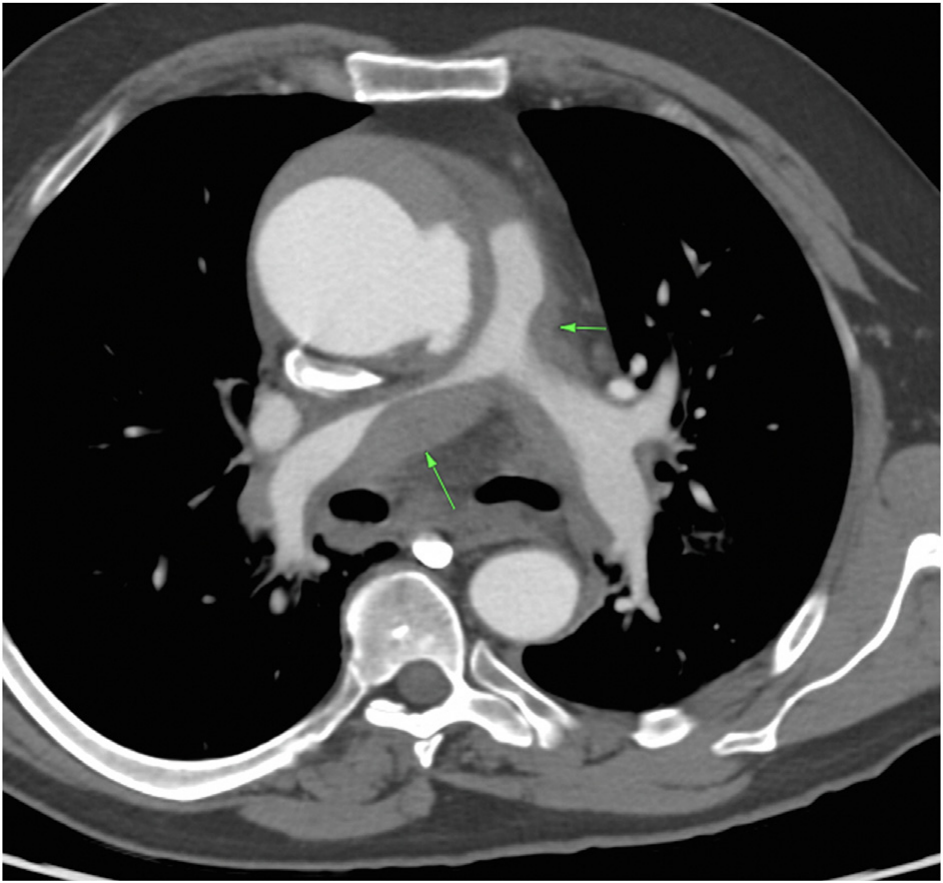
Axial contrast-enhanced computed tomography image demonstrating a large volume of high-attenuation material tracking along the central pulmonary arteries, consistent with intramural hematoma. There is moderate narrowing of the main pulmonary artery and severe narrowing of the mid right pulmonary artery (green arrows). This may be easily misinterpreted as a saddle pulmonary embolism if proximal aortic disease is missed.
